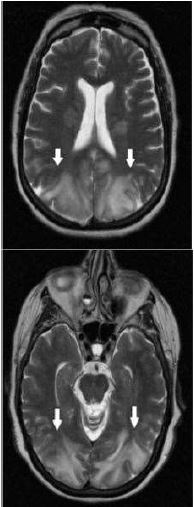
Magnetic resonance imaging brain obtained on postoperative day eight demonstrating T2-weighted hyperintensities (arrows) localized to white matter of bilateral posterior cerebral cortex (bottom) and cerebellum (top).
